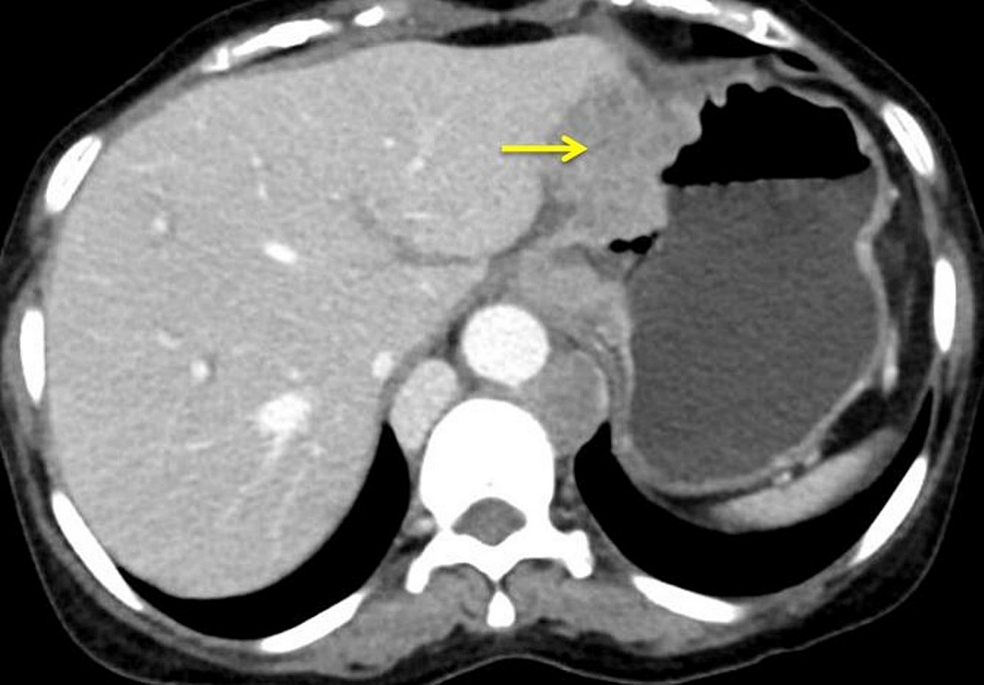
Contrast-enhanced computed tomography (axial view) showing growth extending to the lesser curvature (arrow).
